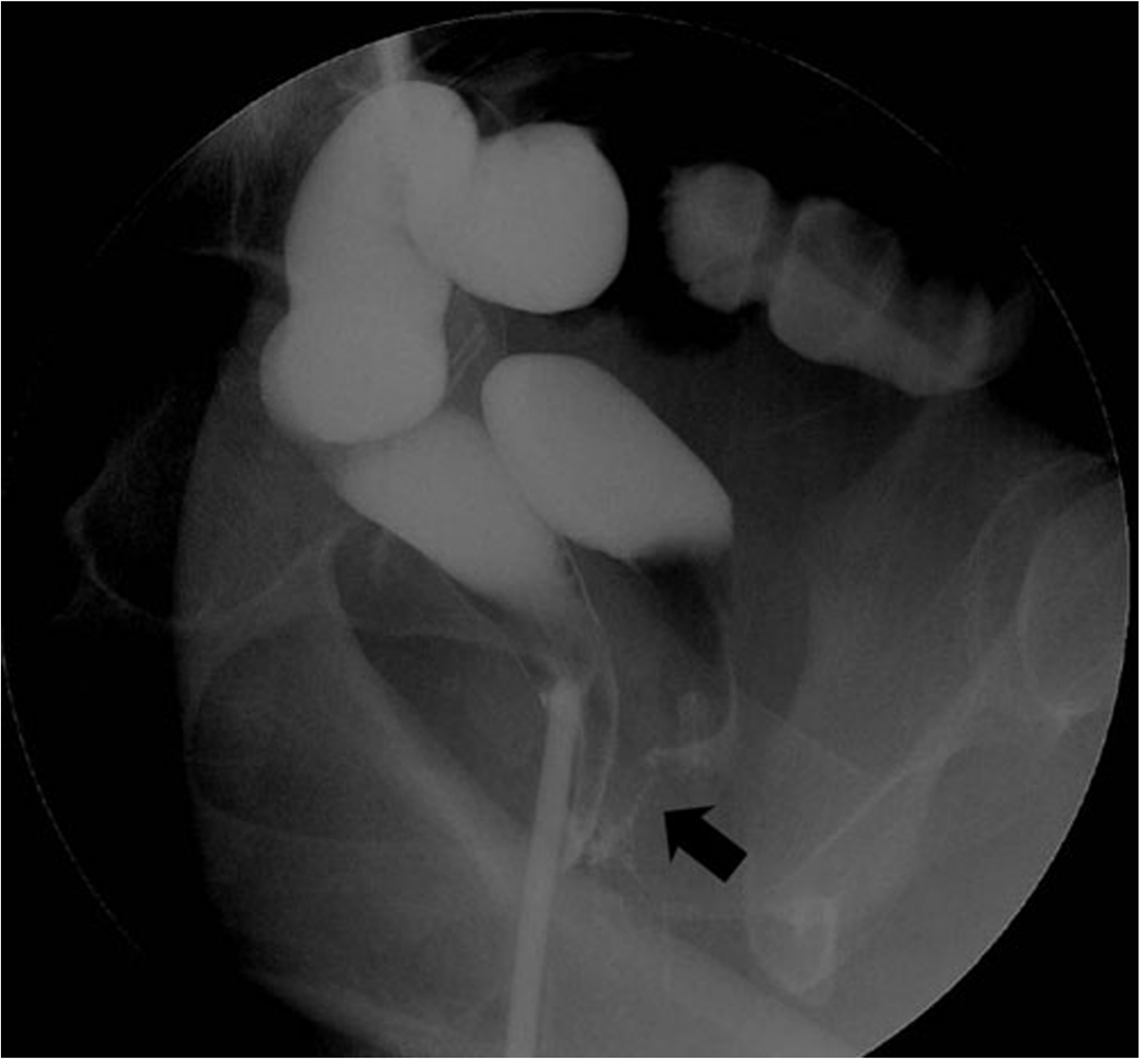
Contrast radiography. Black arrow shows rectovaginal fistula.
